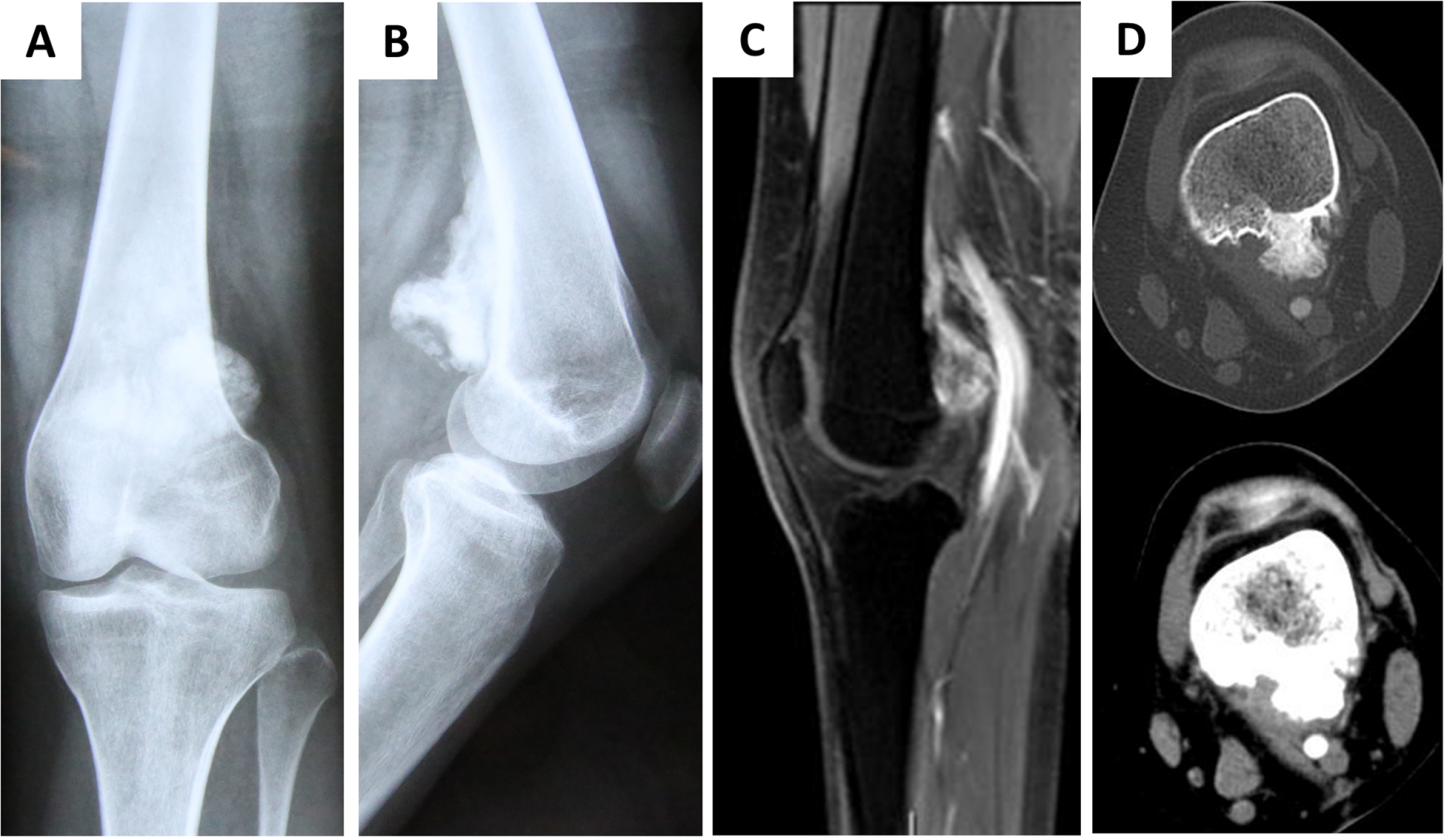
Image features of chondrosarcoma in the metaphysis of the distal femur. Nineteen-year-old female patient with chondrosarcoma. a, b X-ray indicated a large osteogenic bone lesion at the posterior femur of the left leg. c MRI enhanced scan showing the lesion was closely adjacent to the popliteal vessels. d Cross-section of CT scan demonstrated that the lesion was close next to the vessels
